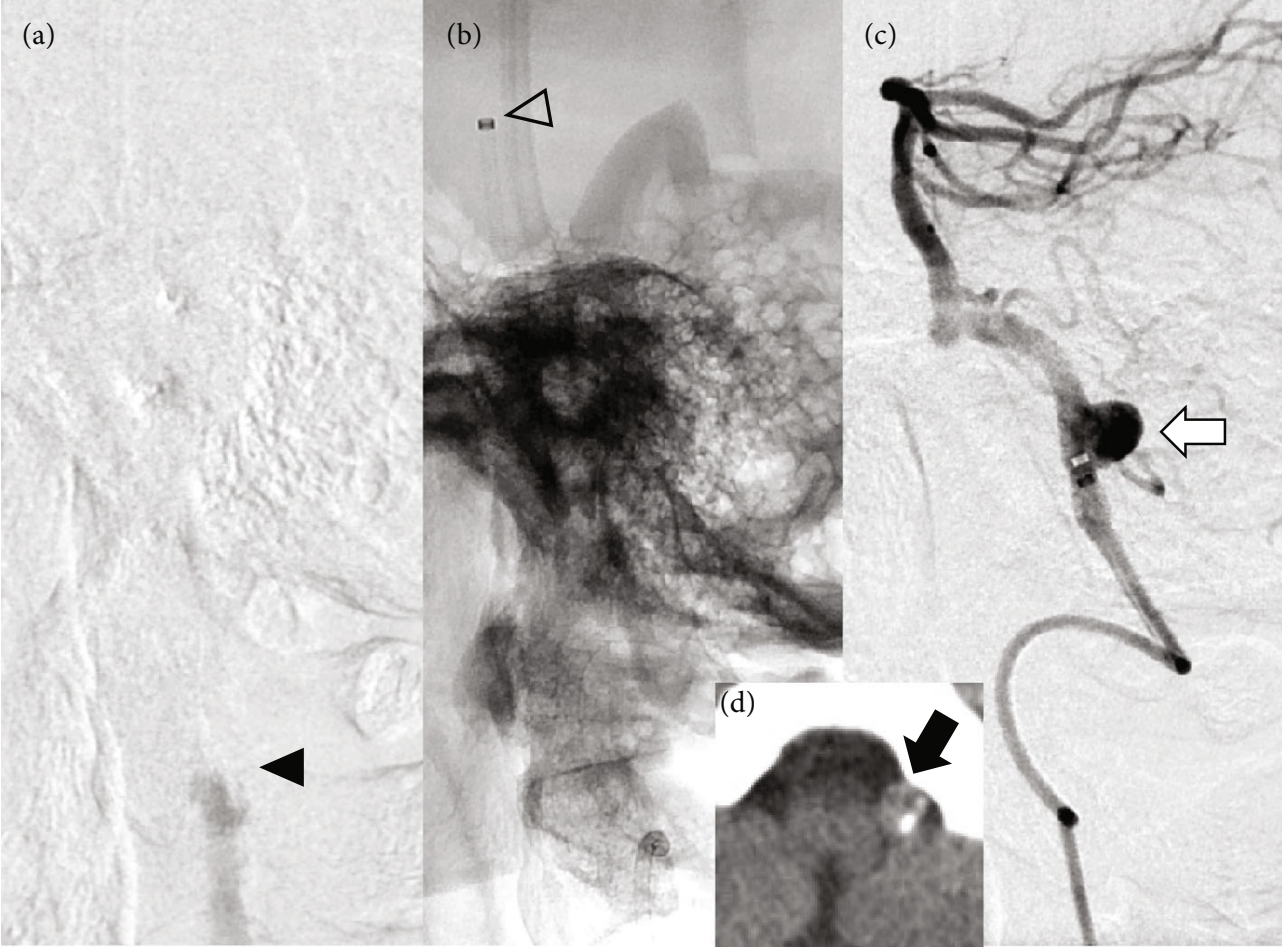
Case 6. (a) Lateral view of left vertebral angiography showing occlusion of the left vertebral artery (black arrowhead). (b) White arrowheads showing the tip of the aspiration catheter navigated to the top of the basilar artery. (c) After aspiration thrombectomy, the saccular aneurysm at the VA-PICA bifurcation was visualized (white arrow). (d) Suspected presence of an aneurysm of the left VA based on preoperative CT findings (black arrow). VA-PICA, vertebral artery-posterior inferior cerebellar artery; VA, vertebral artery; CT, computed tomography.
